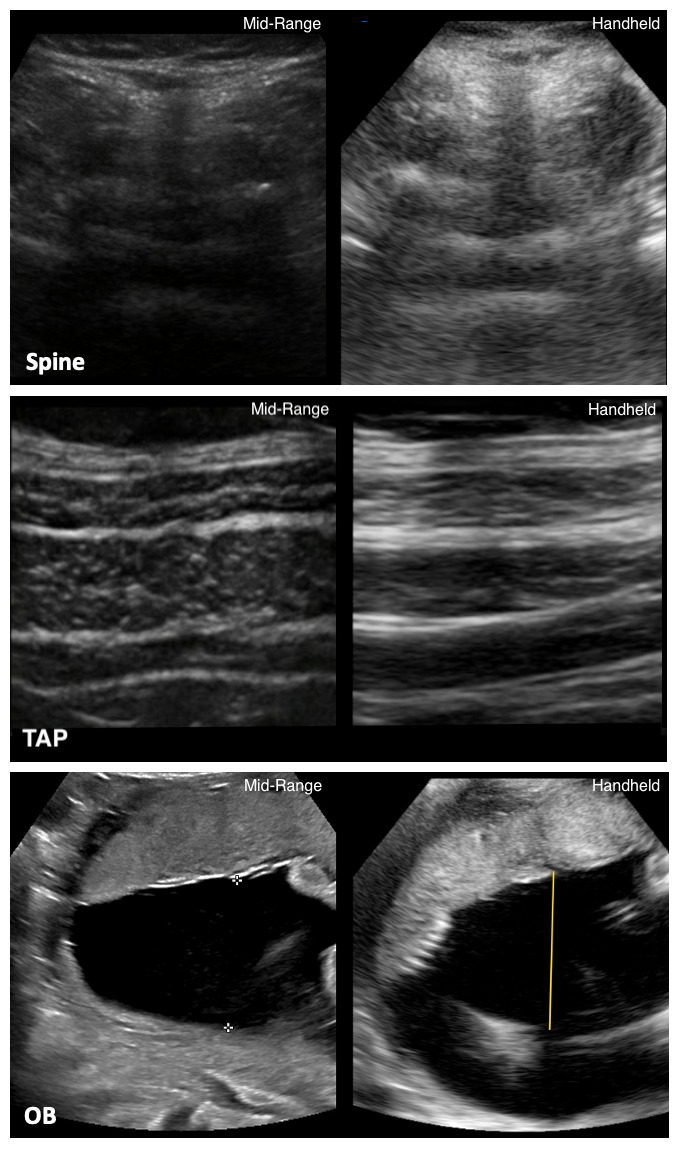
Examples of paired images. Images were unlabeled, cropped, masked, deidentified, and presented in randomized pairs to experienced sonographers for grading. Images were presented by group (Sp, TAP and OB) and graded on a Likert scale from 1-10 on image resolution (RES), detail (DET) and quality (IQ).
